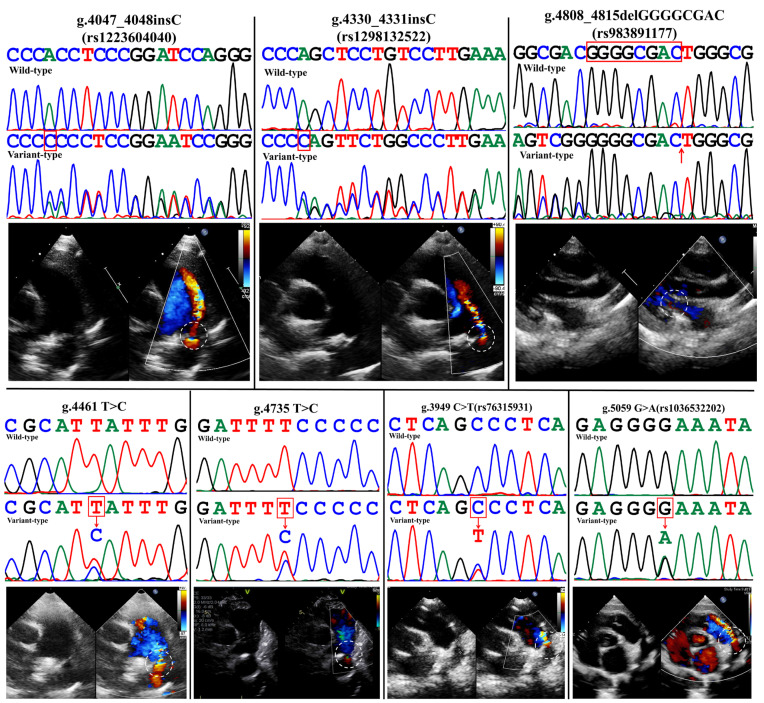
Sanger sequencing results from the 3’ end to the 5’ end and the relative transcriptional activity of CITED2 gene promoter. Sanger sequencing map of CITED2 gene promoter variation and echocardiography of PDA patients. The variants only discovered in CITED2 promoter of PDA patients and verified by cell function in this study are shown as g.3949C > T(rs76315931), g.4047_4048insC(rs1223604040), g.4330_4331insC(rs1298132522), g.4461T > C, g.4735T > C, g.4808_4815delGGGGCGAC(rs983891177), and g.5059G > A(rs1308068829). Top panel: wild type. Bottom panel: hybrid variant, followed by Doppler echocardiography screenshot of the corresponding PDA patient. The red arrow in the panel of g.4808_4815delGGGGCGAC(rs983891177) indicates the position of the missing fragment “GGGGCGAC”. The DNAMAN comparison results for variant rs983891177 are presented in the Supplementary Material placed as Figure S2. The white dashed circle marks the position of the PDA. rs number: a systematic identifier applied to human genomics to locate individual positions in genotype analysis, also known as SNPs. The two variants not labeled with rs numbers are novel variants discovered for the first time in this study.
